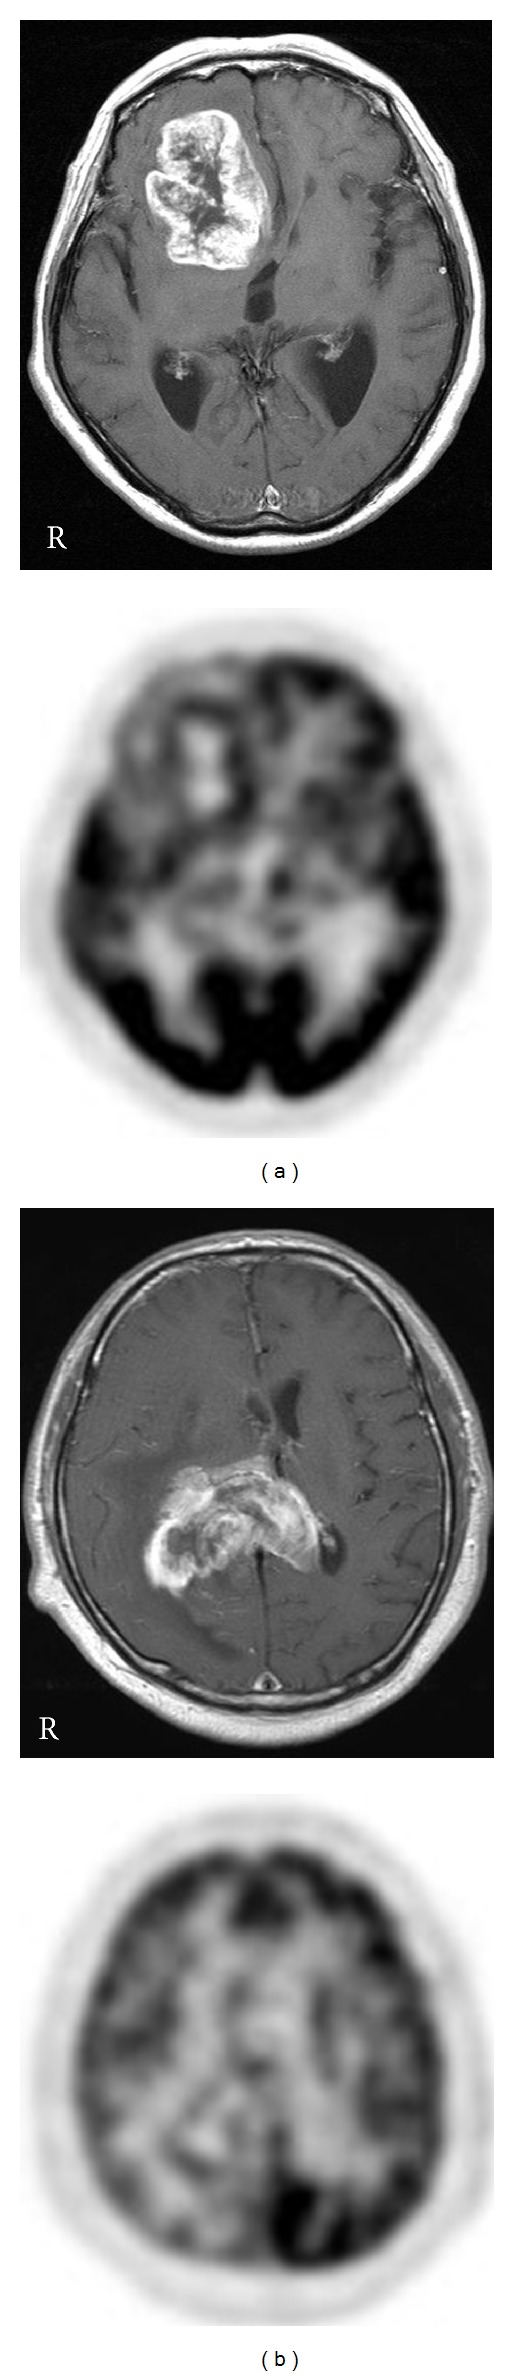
Contrast-enhanced T1-weighted MR (upper) and ^18^F-FDG PET (lower) images in GB patients. MR images show a heterogeneous ring-like enhanced lesion in the right frontal lobe (a) and the splenium (b). PET images show a mild ring-like ^18^F-FDG uptake in the lesions.
